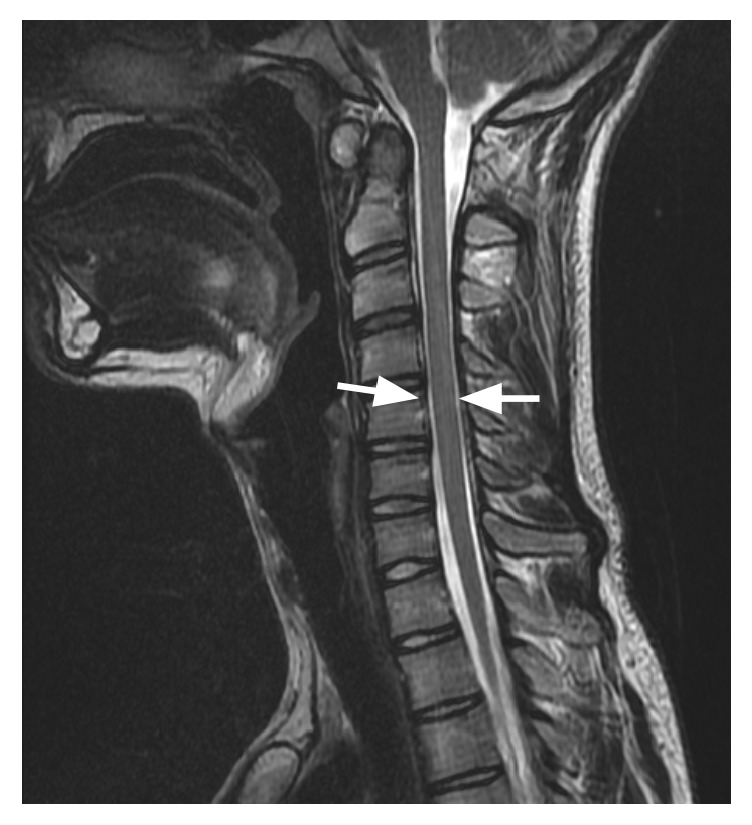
Cervical MRI image showing spinal canal caliber on the lower end of normal limits (white arrows).
